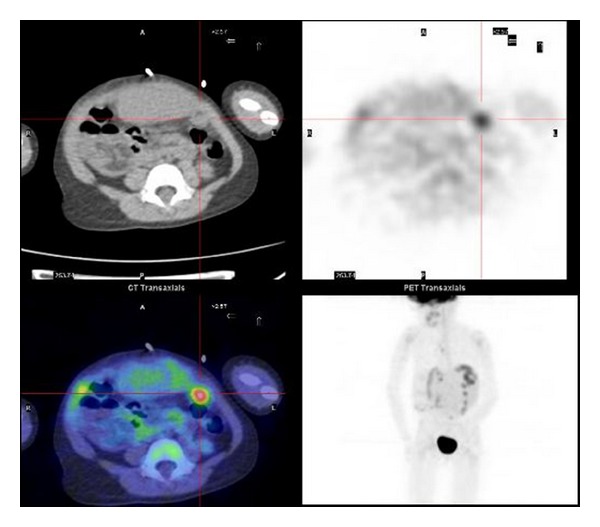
Peritoneal recurrence of hepatoblastoma. ^18^F-FDG-PET/CT transverse images show a peritoneal nodular mass with abnormal FDG uptake suspicious for recurrent hepatoblastoma. The biopsy confirmed the diagnosis.
